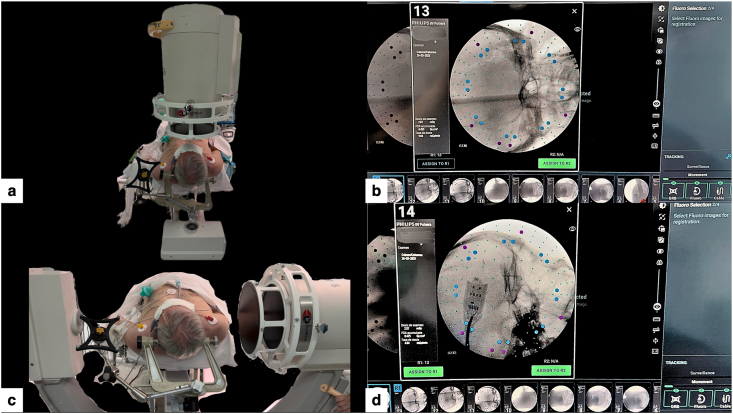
CT-to-fluoroscopy acquisition for ExcelsiusGPS registration. (a) Intraoperative setup with the C-arm positioned for orthogonal anteroposterior cranial fluoroscopy, with the dynamic reference base (DRB) in view. (b) Anteroposterior fluoroscopic image selected for registration, showing clear visualization of skull base bony landmarks. (c) C-arm positioned for true lateral cranial fluoroscopy while maintaining an unobstructed line-of-sight to the DRB. d) Lateral fluoroscopic image selected for registration, providing complementary landmarks for subsequent CT-to-fluoro “Merge Images” alignment.
